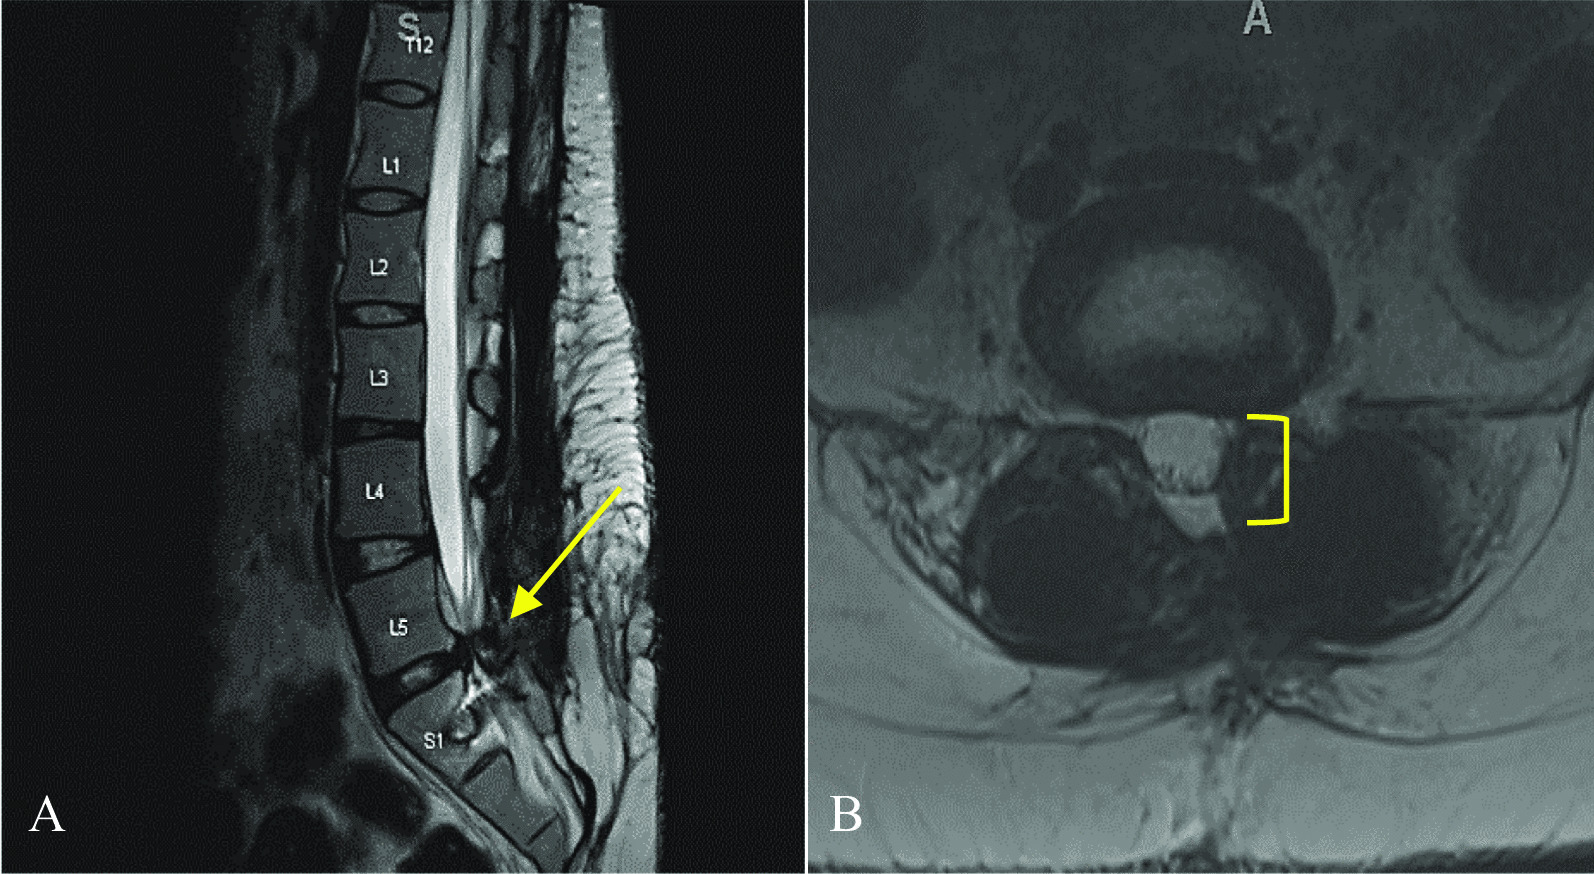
Follow-up images obtained 1 year following redo lumbar decompression and fusion surgery and subsequent washout. a T2-weighted sagittal MRI at 1-year postoperative, demonstrating decompression of L4–L5 level but with increased stenosis at L5–S1 (arrow). b T2-weighted axial MRI showing decreased stenosis (bracket) at the L4–L5 level
